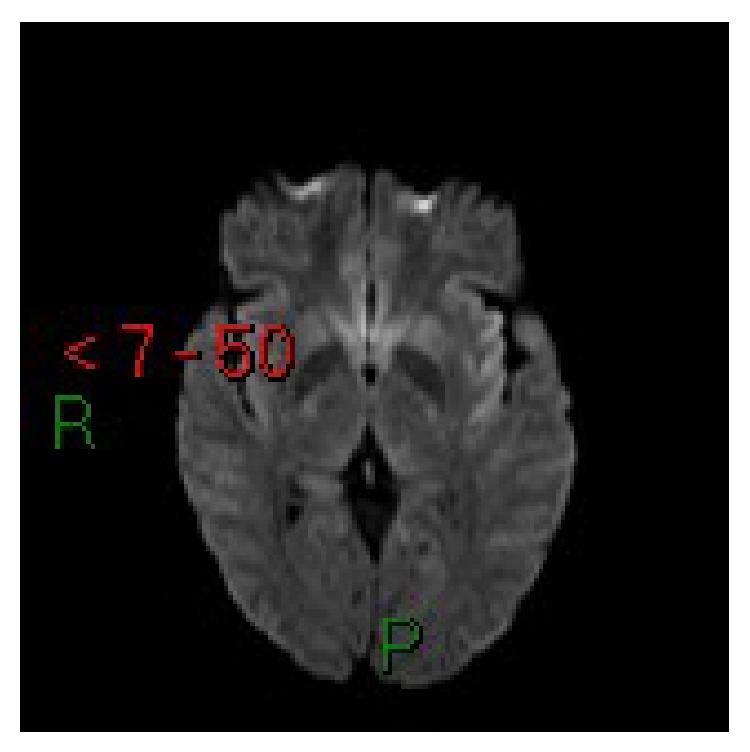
Case 3. Axial diffusion-weighted MRI: lesions showed a slight signal elevation.
